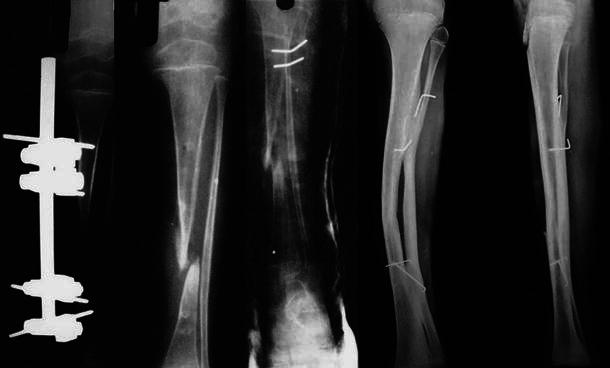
Radiographs of 7-year-old body showing pre-operative X-ray with external fixator in situ, tibialization of fibula and radiographic union at final follow-up at 9 years post-surgery showing good consolidation and hypertrophy of fibula
